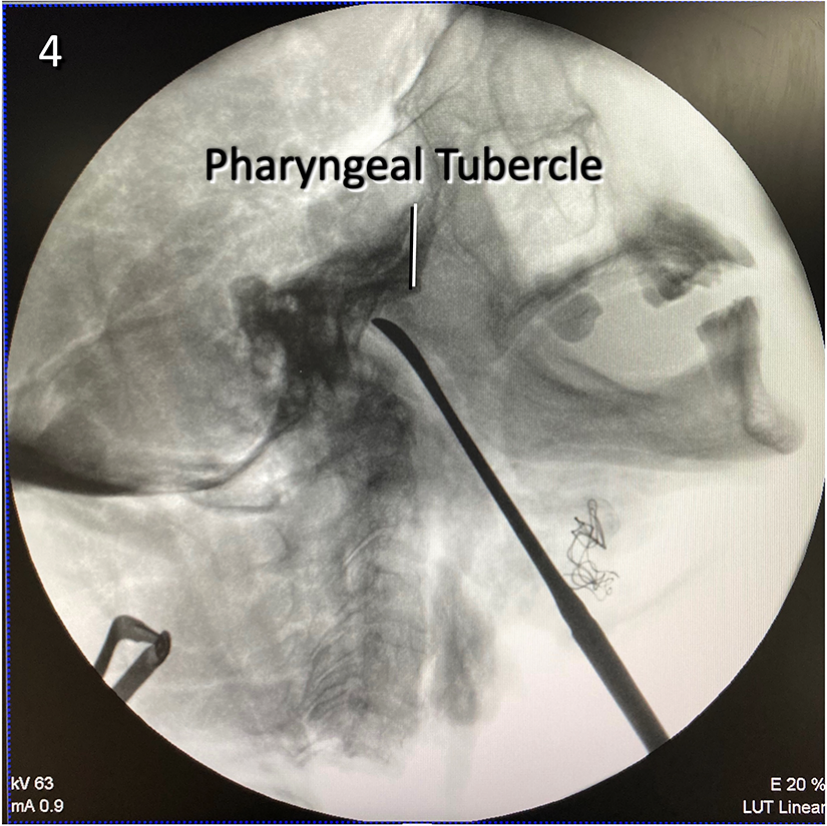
Lateral x-ray view of the retropharyngeal corridor used to reach the craniocervical junction. The tip of the dissector points to the lower clivus.
